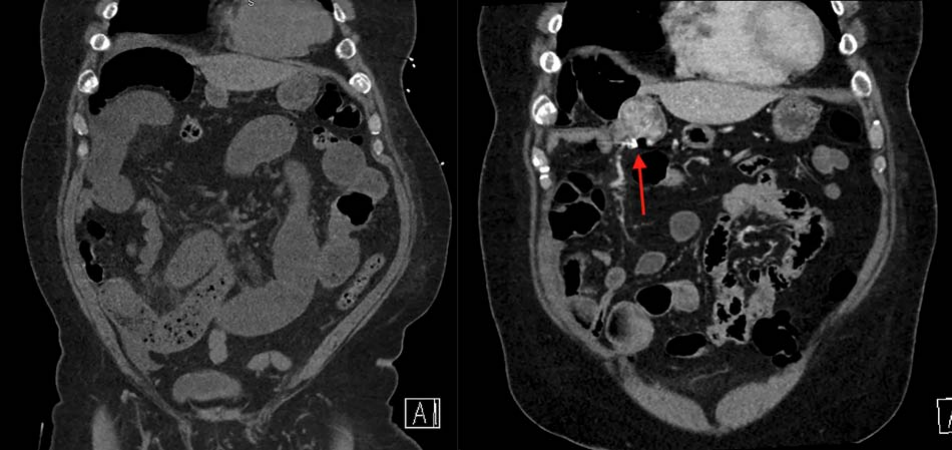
On the left is the initial CT scan non-contrast demonstrating dilated small bowel loops and a transition point in the right lower quadrant. On the right is the CT angiography (CTA), performed a week later, showing a mass and pill cam in the right upper quadrant.
